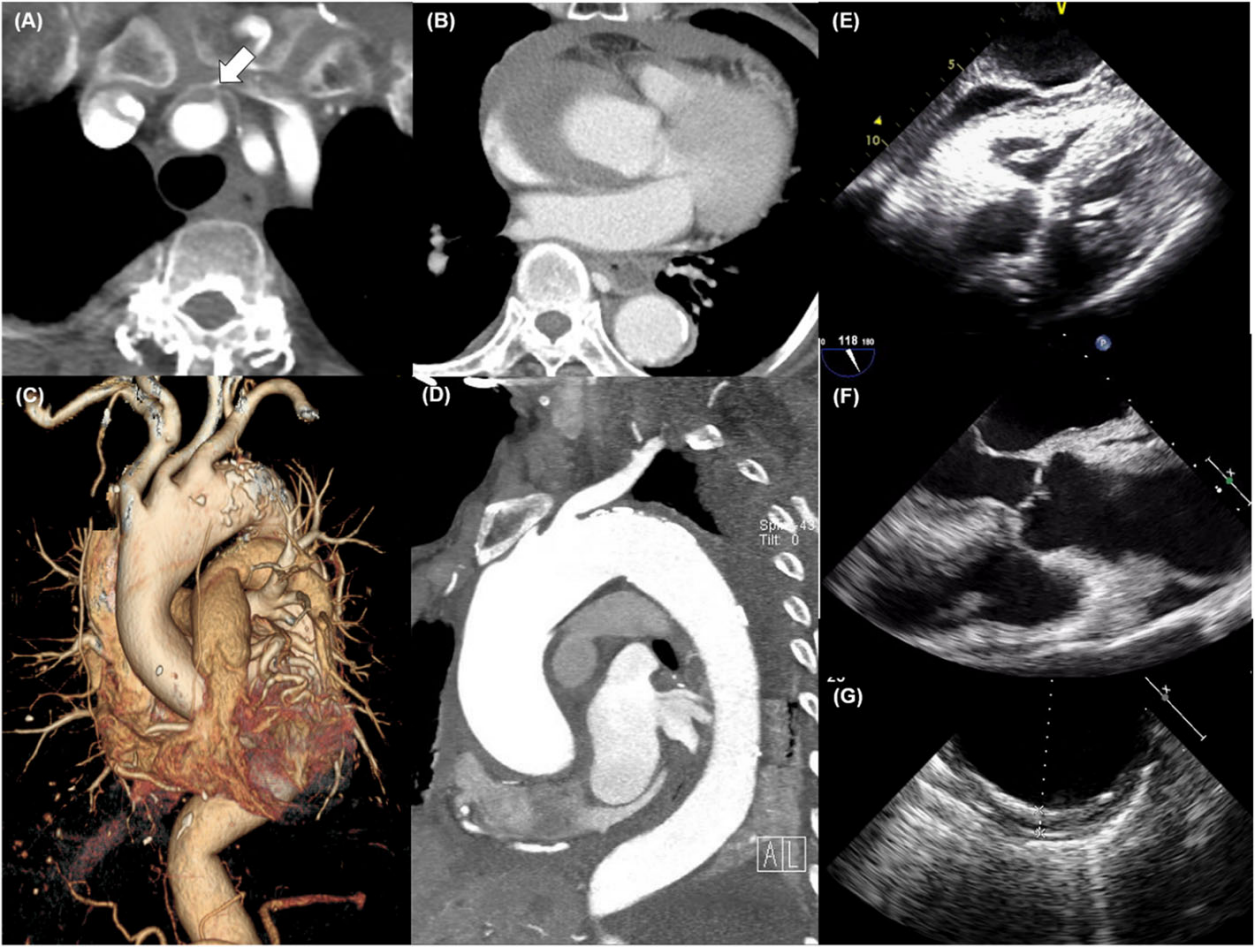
a-d Computed tomography (CT) angiography and e transthoracic and f-g transesophageal echocardiography (TEE) scans; a-b Aorta CT angiography, axial plane. a The site of entry tear is suspected to be located in the proximal brachiocephalic artery (white arrow) but a dissection flap cannot be clearly observed; b This aortic dissection (AD) mimics an intramural hematoma (IMH) retrogradely extending to the sinuses of Valsalva resulting in pericardial effusion; c Three-dimensional reconstructed image and d Sagittal oblique reconstruction image of aorta CT angiography shows the extent of AD mimicking an IMH extending anterogradely to the proximal descending thoracic aorta; e subcostal view of transthoracic echocardiogram reveals newly developed, moderate amount of pericardial effusion; f TEE (135 degree long axis view) showed no definite dissection flap and intramural hematoma in the aortic root. g TEE (0 degree short axis view) revealed an IMH extending anterogradely to the proximal descending thoracic aorta
